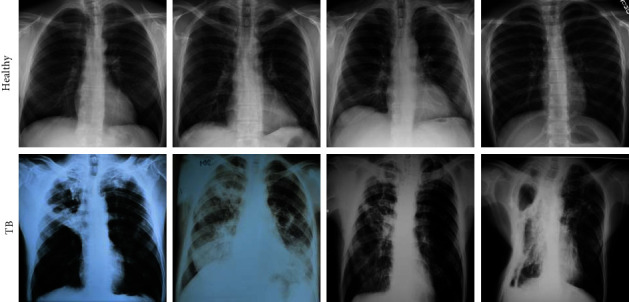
Sample X-ray images of healthy/TB class.
